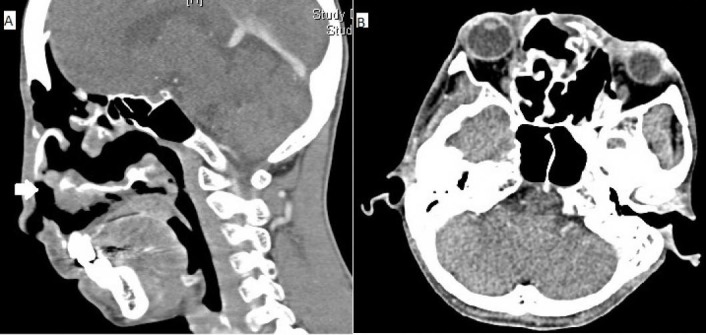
Post-treatment CT performed six weeks post RT (A) Sagittal section showing near complete resolution of the solid components of the tumor, with significant improvement in the nasal cavity (white arrow). (B) Axial section showing medialization of the orbits. Computed tomography (CT); Radiotherapy (RT).
